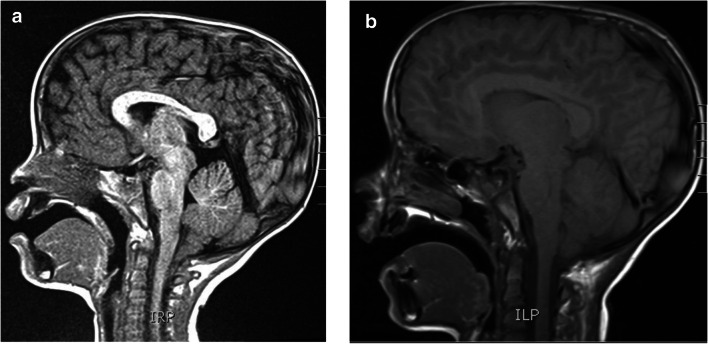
a Sagittal view MRI of the patient at 2 months of age. As can be seen above, the cerebellar tonsils protrude into the foramen magnum at approximately 1.3 cm, demonstrating a Chiari I malformation sagittal view MRI of the patient at eight years of age. b There is complete resolution of the Chiari I malformation, the formation of more normal cerebellar tonsils, global CSF flow, and overall decompression
